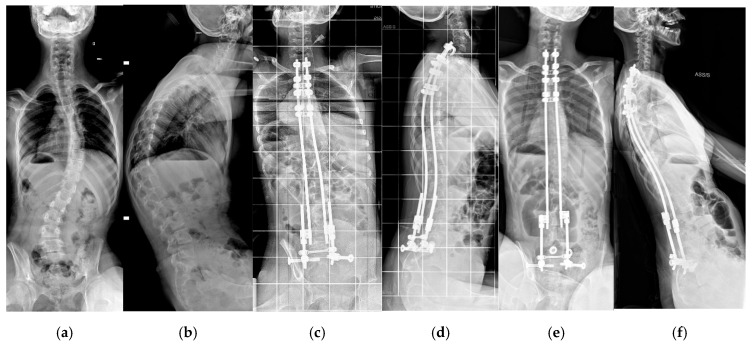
A 12-year-old girl operated on with the self-expanding rods: (a,b) preoperative AP and lateral X-rays; (c,d) in postoperative X-rays (e,f) at 3 years follow-up, we observed spontaneous and complete rod expansion thanks to bone growth.
